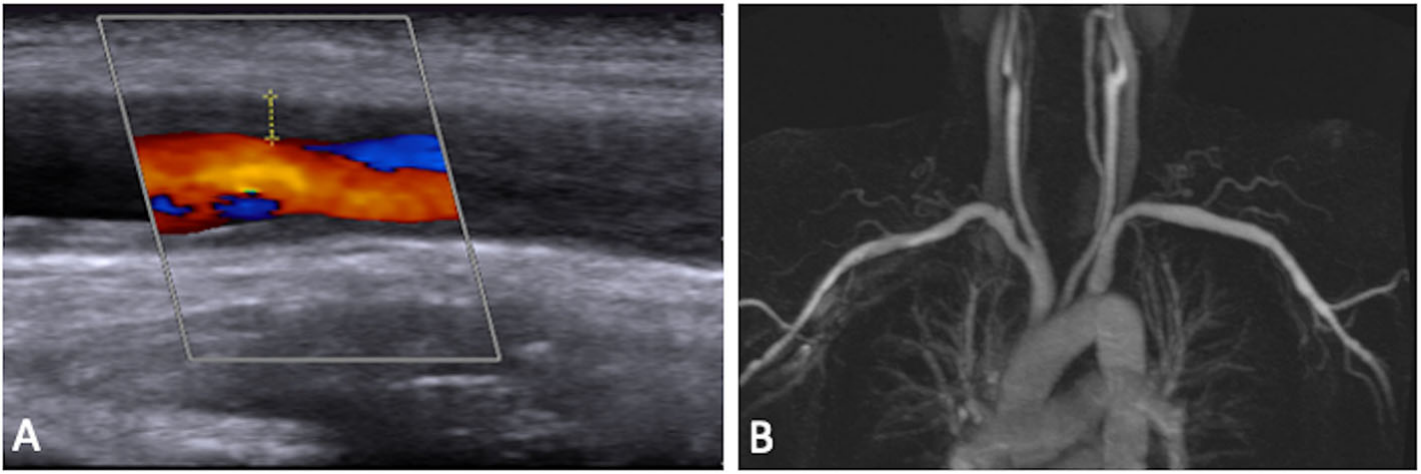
Ultrasound images (a) revealing increased intima-media thickness of the right axillary artery, measured at 1.8 mm on the upper vessel wall, compared to magnetic resonance angiography (b) in the same patient, which did not reveal evidence of vasculitis in the same vessel
